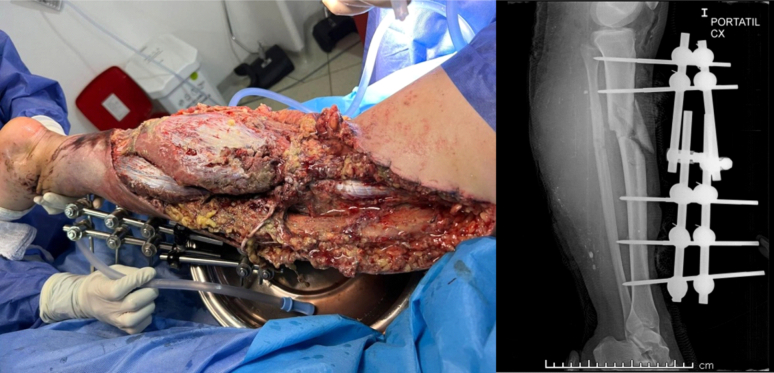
External fixator, lateral gastrocnemius vicinity flap and postoperative x-ray with acceptable fragment alignment.
